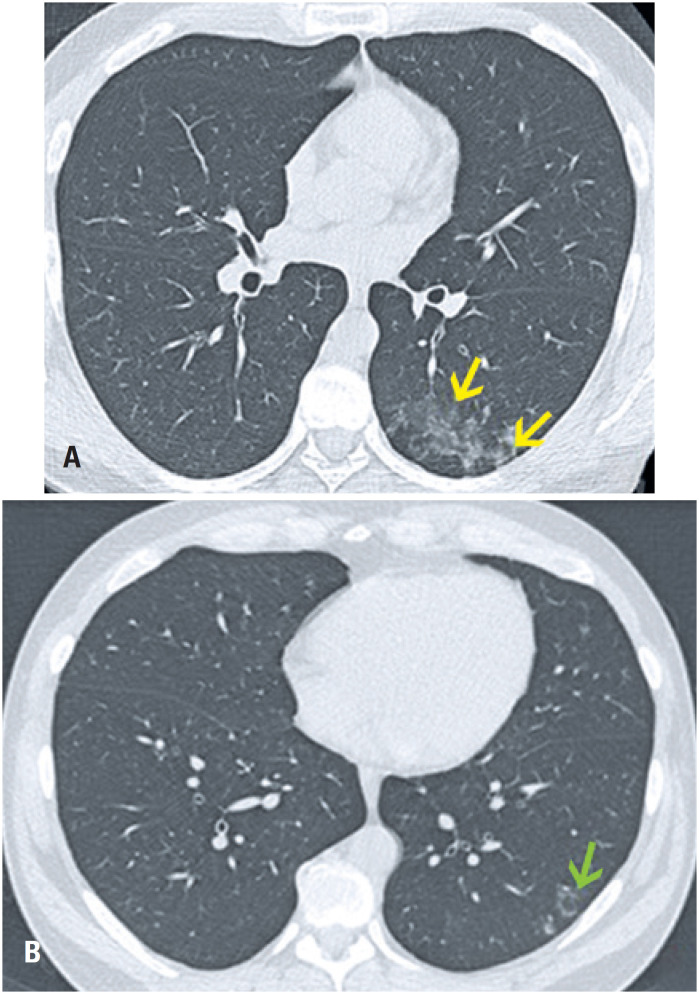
A 44-year-old male smoker had the initial exam (A) For a medical check-up. After 10 months, he had another exam (B) Due to cough and malaise. (A) Computed tomography with axial section of the pulmonary window: centrilobular ground-glass opacities, sometimes confluent, sparsely distributed in the lower left lobe; (B) Computed tomography with axial section of the pulmonary window: density reduction of centrilobular ground-glass opacities - some showing an reversed halo sign (green arrow)
